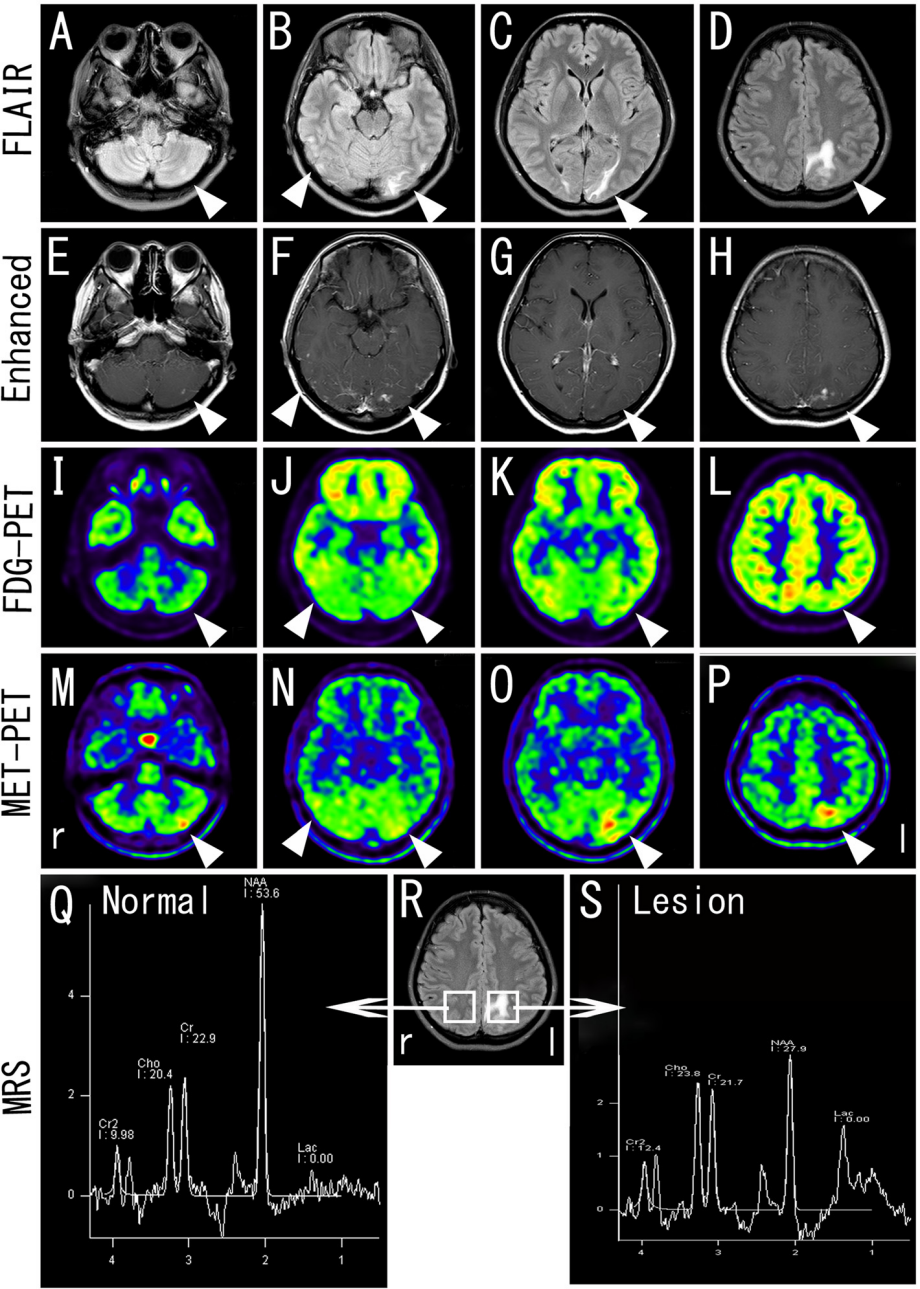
Brain MRI, PET-CT, and MRS findings at 4 months after coiling. 2-deoxy-2-[F-18]fluoro-D-glucose (FDG) and ^11^C-methionine (MET) PET studies showed that glucose uptake in the lesion with hyperintense areas by FLAIR image (A-D, arrowheads) and enhanced areas by gadolinium-enhanced MRI (E-H, arrowheads) increased slightly or remained at the baseline level (I-L, arrowheads), whereas methionine uptake increased (M-P, arrowheads). ^1^H-MRS showed a slight increase of the choline (cho) peak and a marked decrease of the N-acetylaspartate (NAA) peak with an elevated lactate peak in the lesion (Q-S).
